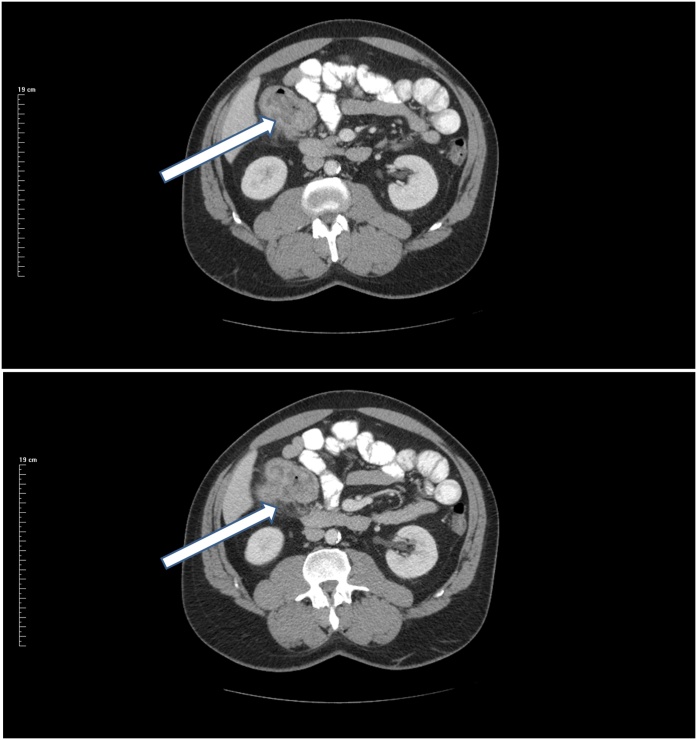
(Top) CT slice through a primary ascending colon adenocarcinoma. The greatest dimension of the mass was 8 cm. The mass touches the visceral surface of the right lobe of the liver and the second portion of the duodenum. (Bottom) CT slice shows stranding of the fat posteriorly suggesting a localized perforation.
